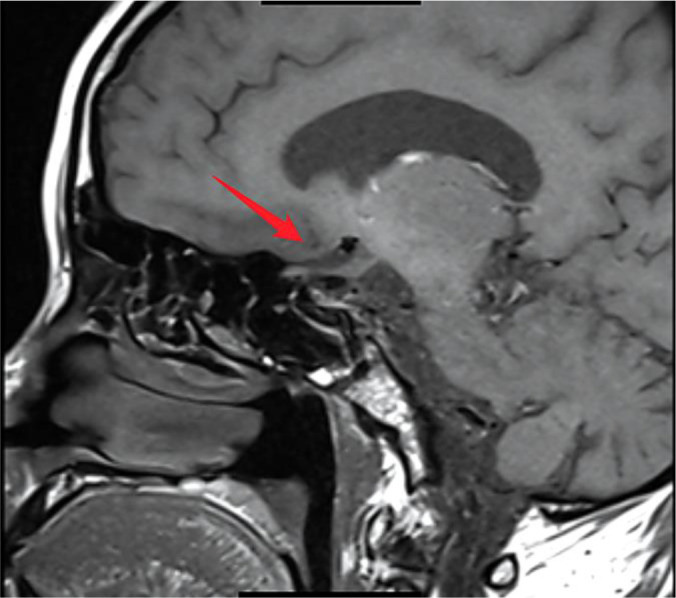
Post-operative pituitary MRI (sagittal view). A 3.0T contrast-enhanced T1-weighted sagittal image demonstrating significant post-operative changes in the sellar and suprasellar region. The arrow indicates abnormal soft tissue in the suprasellar area, consistent with post-surgical alterations following craniopharyngioma resection.
